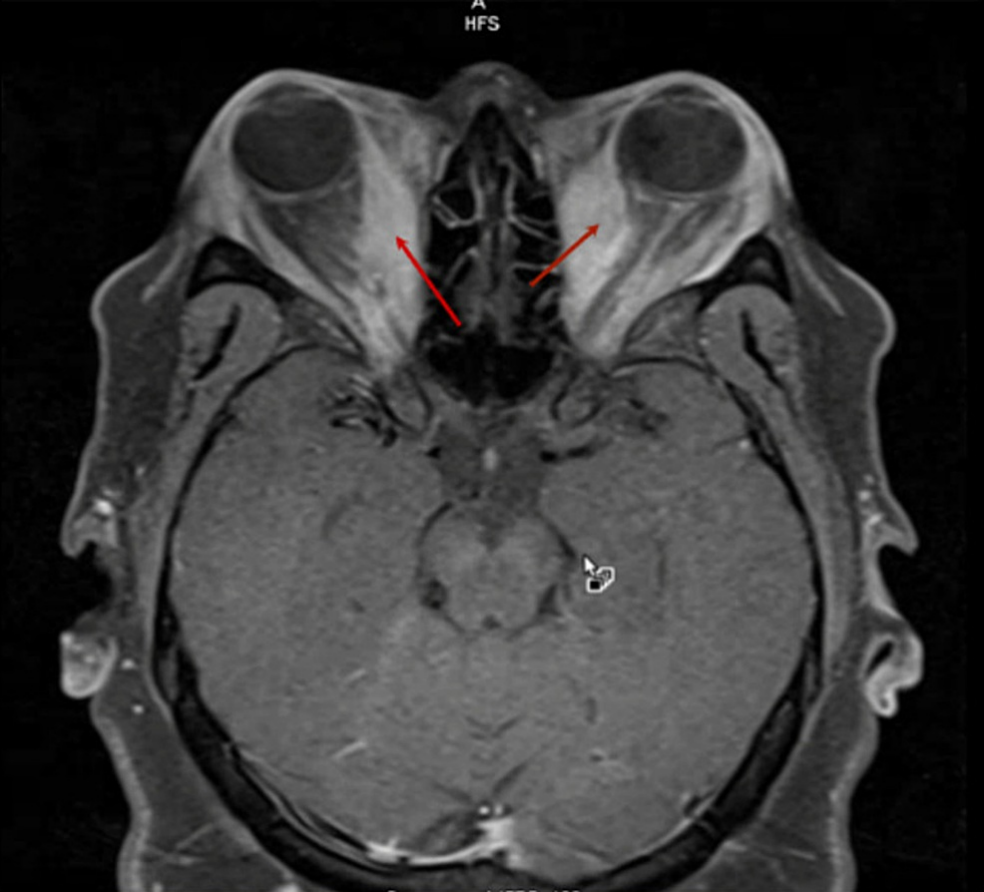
Orbital MRI (axial view) showing significant enlargement of extra-ocular muscles (bilateral medial recti indicated by red arrows), giving a sign known as "Coca-cola bottle" appearance. MRI, magnetic resonance imaging
